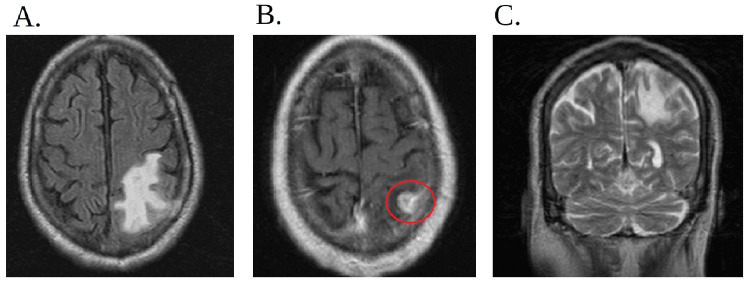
MRI of the brain and head with and without contrast (A) The figure is a transverse view of the MRI of the brain with and without contrast which shows prominent adjacent vasogenic edema. (B) The red circle indicates a 1.5-cm left parietal lobe extra-axial enhancing mass. (C) The figure is a coronal view of the MRI of the brain with and without contrast which shows prominent adjacent vasogenic edema in the left parietal lobe. MRI: magnetic resonance imaging
